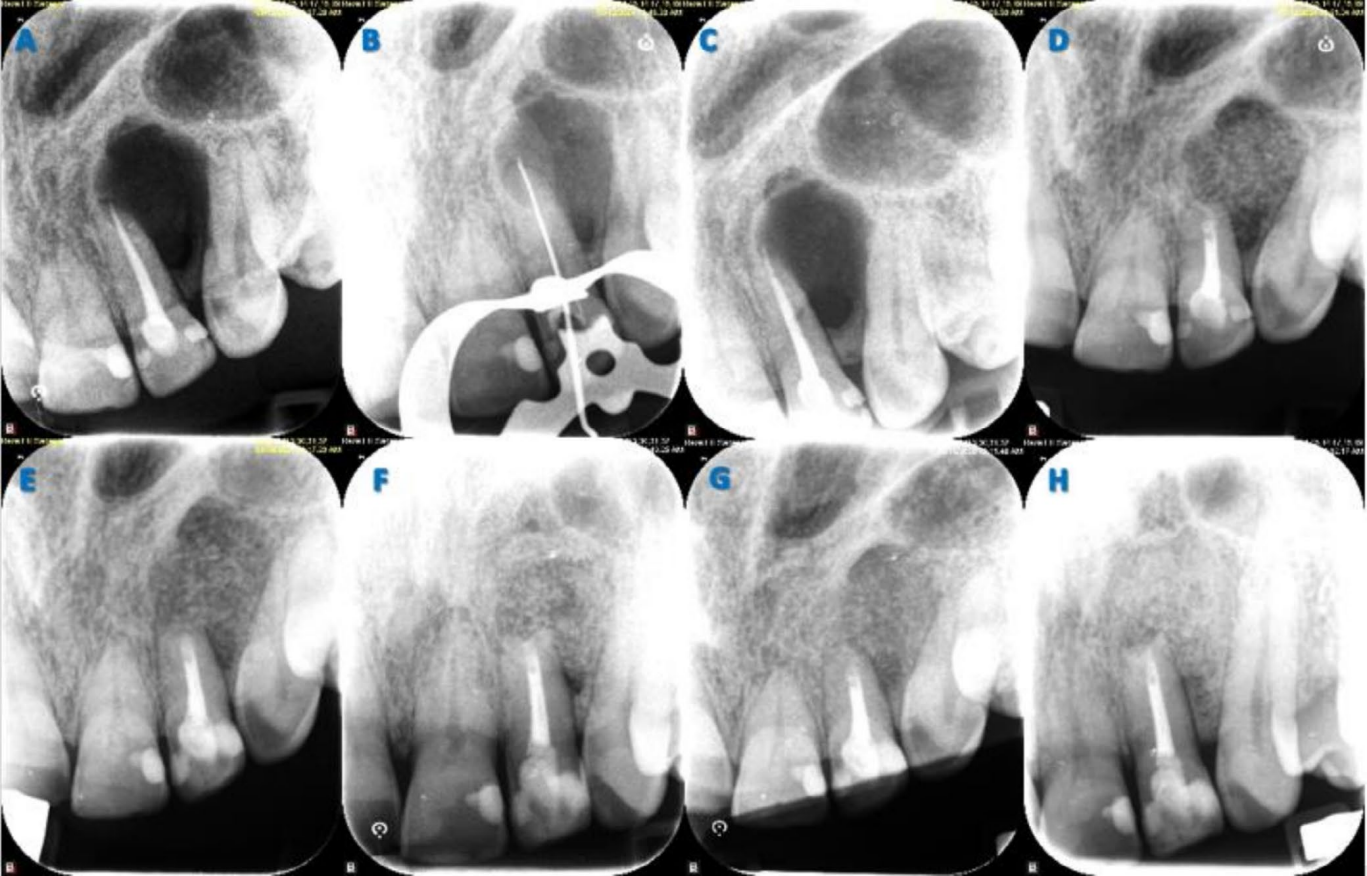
(A) Preoperative periapical radiograph indicating significant periradicular radiolucency; (B) Intraoperative periapical radiograph of length determination; (C) Postoperative periapical radiograph immediately after obturation; (D) Postoperative periapical radiograph immediately after root‐end filling; (E) One‐month follow‐up periapical radiograph; (F) Six‐month follow‐up periapical radiograph; (G) Nine‐month follow‐up periapical radiograph; (H) One year follow‐up periapical radiograph.
